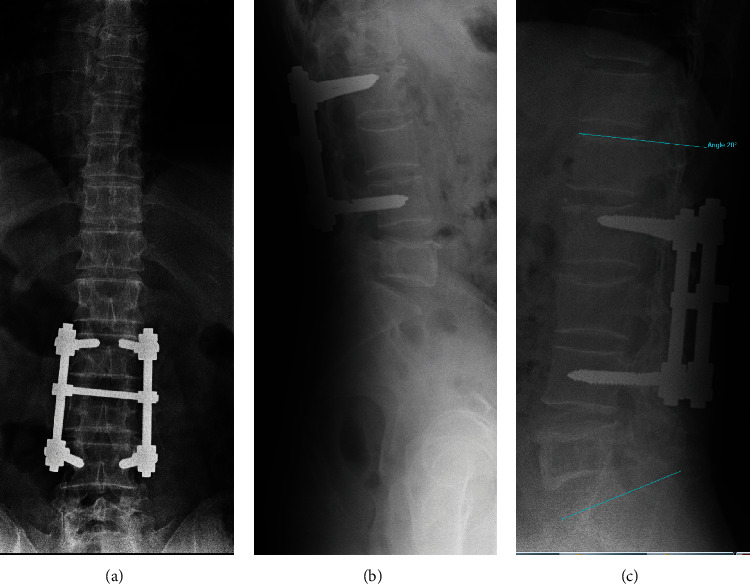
X-ray anteroposterior (a) and lateral preoperative views (b/c) showing previous instrumentation post-fracture intervened years before with lumbar lordosis 28°.
